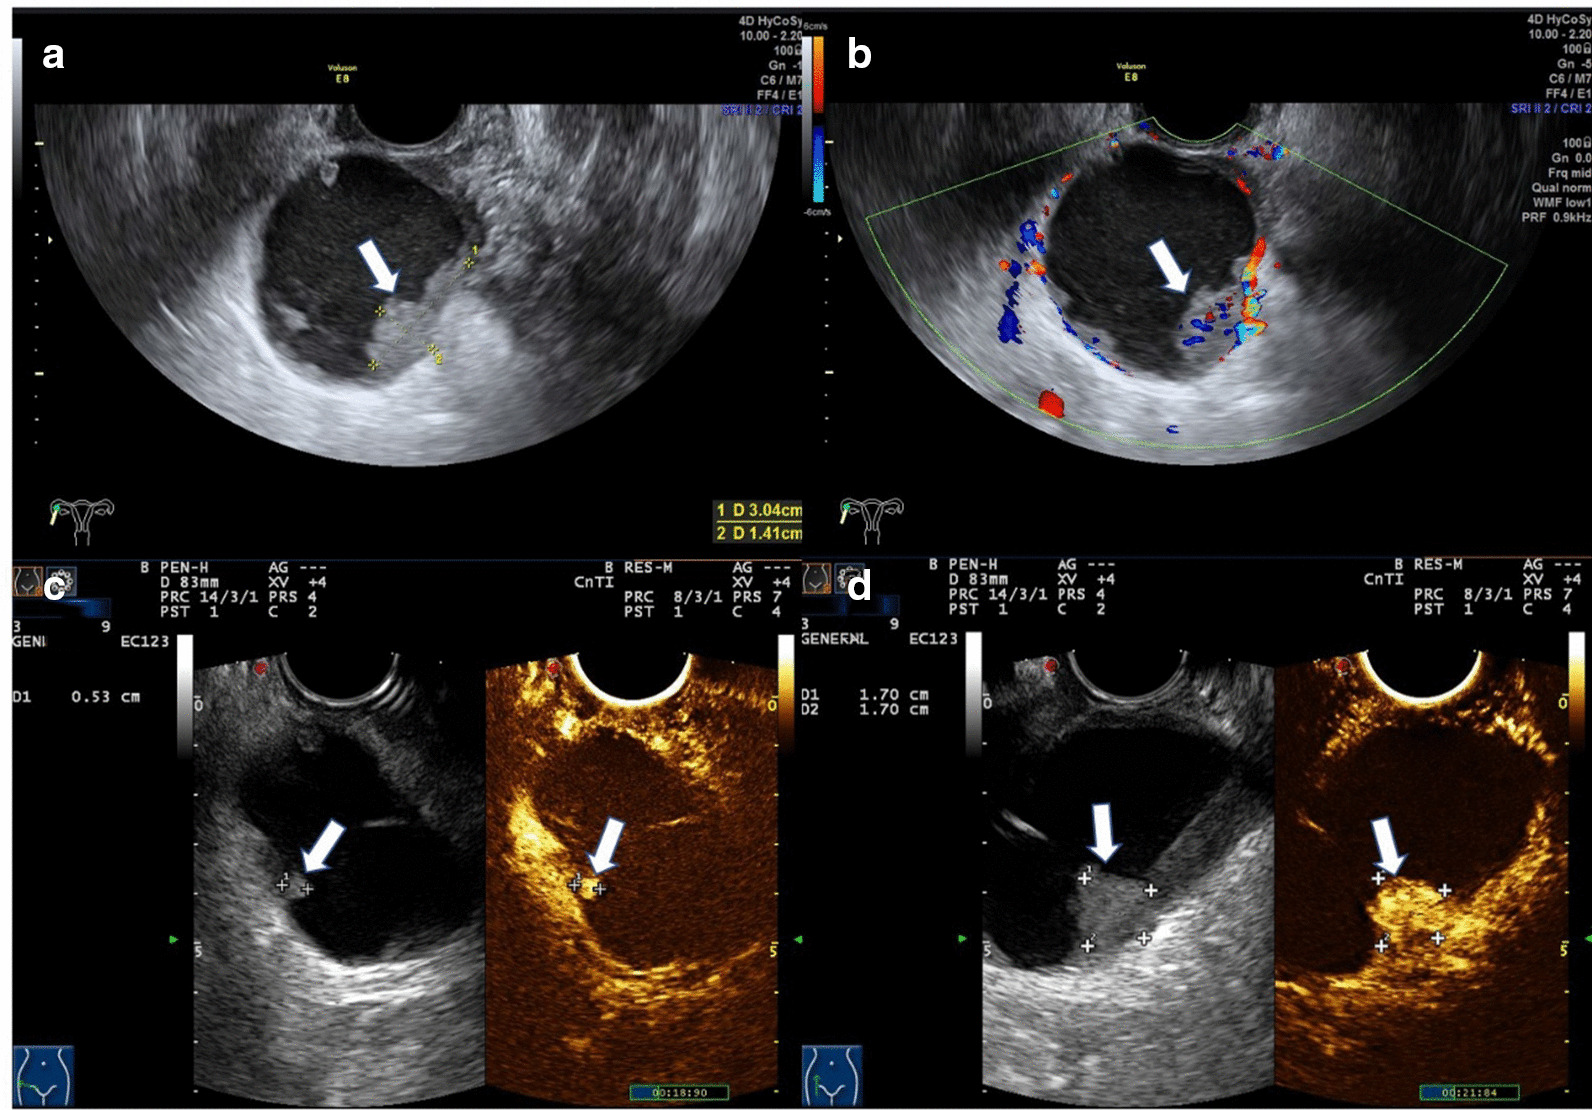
Ultrasound appearance of the PRSA. a Conventional ultrasound shows an unilocular cystic lesion with papillary excrescences measured 3.0 * 1.4 cm (arrow). b Color Doppler image shows the solid papillary projection contains internal flow (arrow). c, d Hyper-enhancement of cystic wall, intracystic septations, and intracystic solid components is found in the arterial phase on CEUS (arrow)
